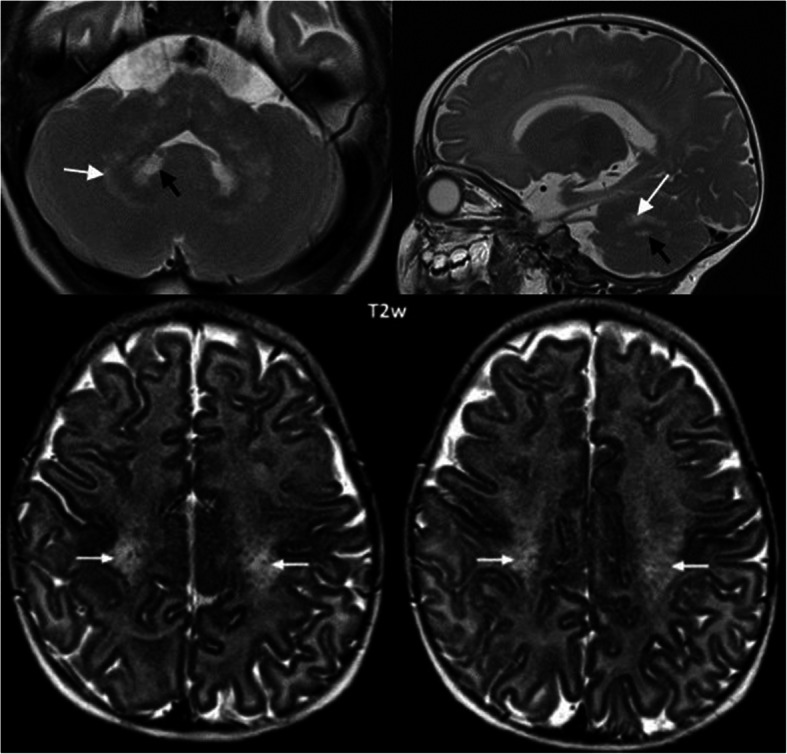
Pattern A1 (early-infantile form). White matter changes prominent in the central region (small white arrows in lower images), cerebellar white matter (white arrows in upper images) and signal changes of the dentate nucleus (black arrows in upper images). T2w images axial lower part, coronal upper left and sagittal upper right. Patient 4 (onset 2 months, age at MRI 5 months).
